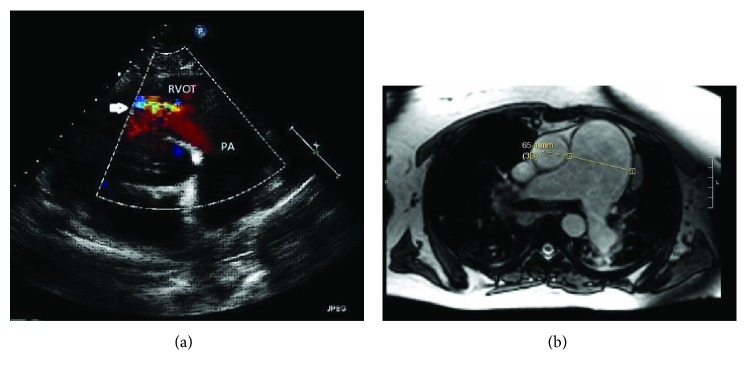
(a) Transthoracic echocardiogram parasternal short axis color Doppler showing severe pulmonic regurgitation (white arrow) and dilated pulmonary artery. (b) Cardiac MRI showing marked aneurysmal enlargement of the main PA.
